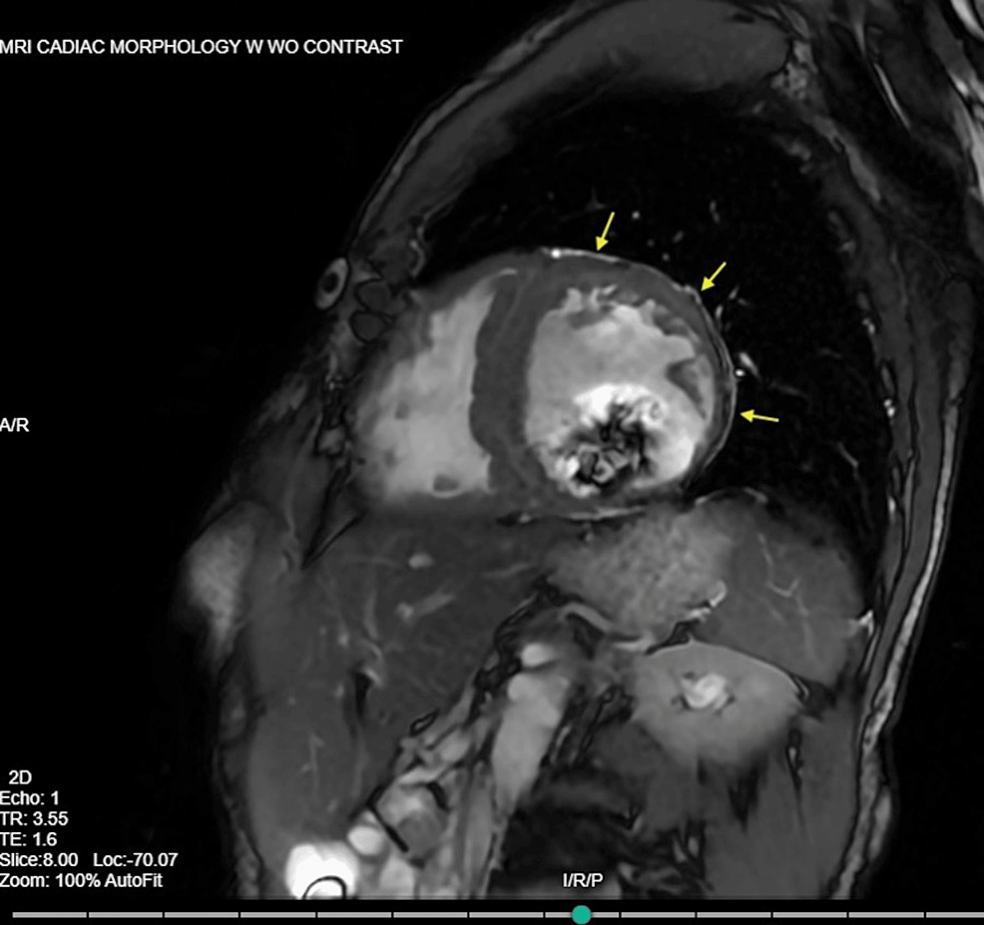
Cardiac Magnetic Resonance Imaging (MRI) showed moderately dilated ventricles and late gadolinium enhancement of the left ventricular free wall indicated by arrows with septal sparing.
